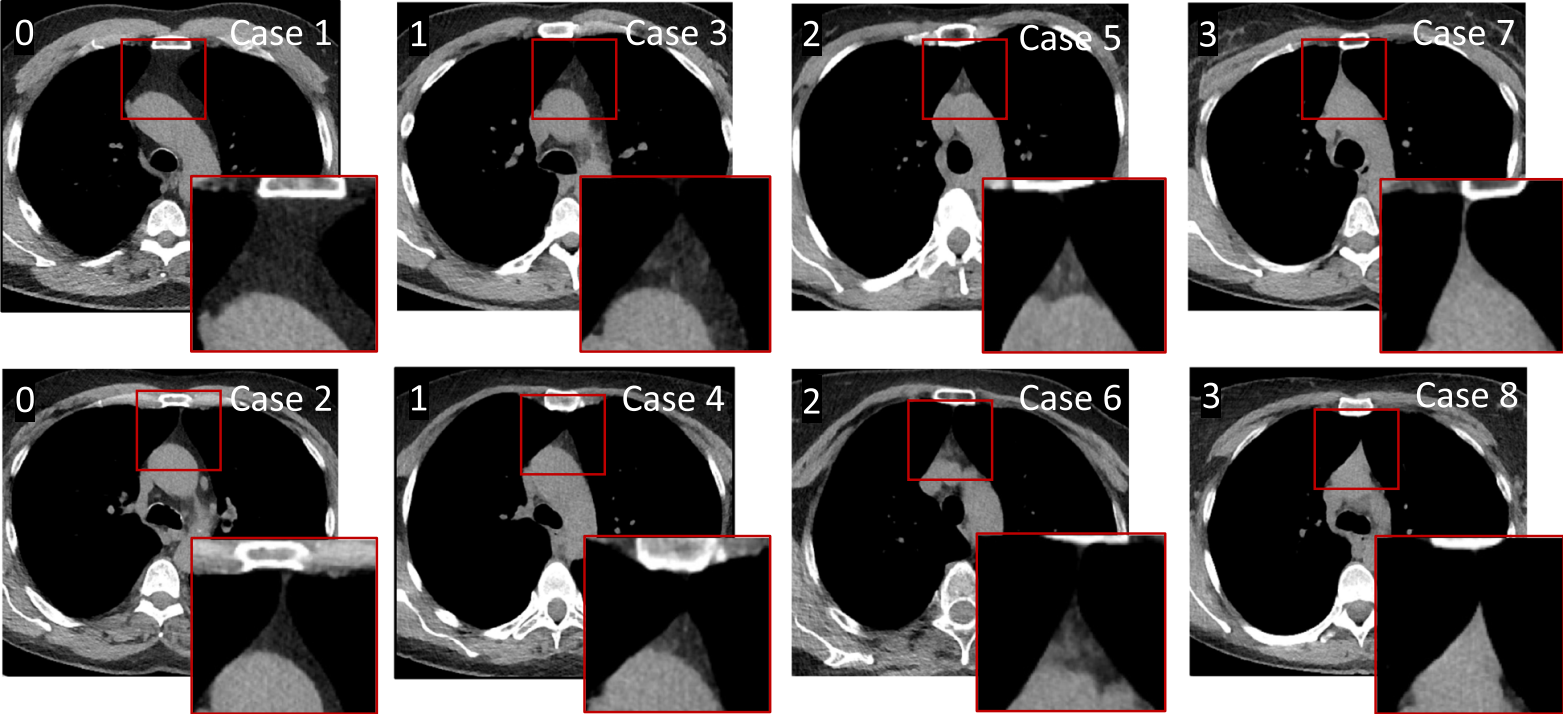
Representative CT images of each thymic score
